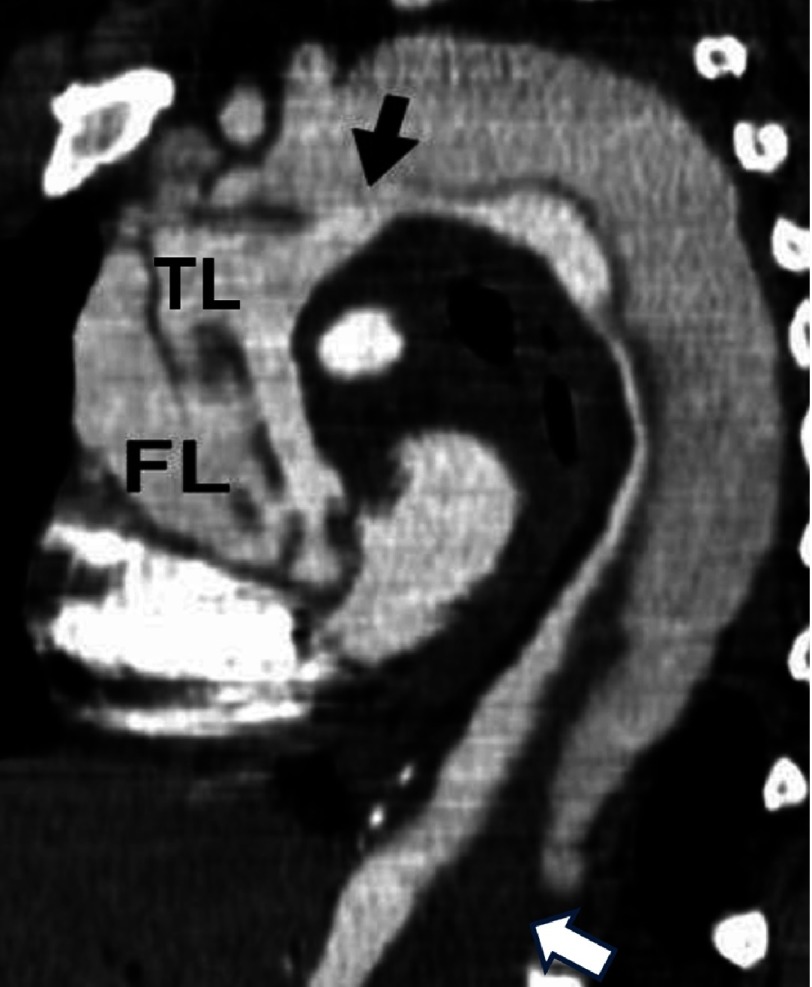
CT shows a type A AAD with a large entry tear in the aortic arch (black arrow) and retrograde dissection to the ascending aorta and antegrade descending thoracic aorta dissection with distal total thrombosis of false lumen (white arrow). Abbreviations: TL= true lumen, FL= false lumen.
